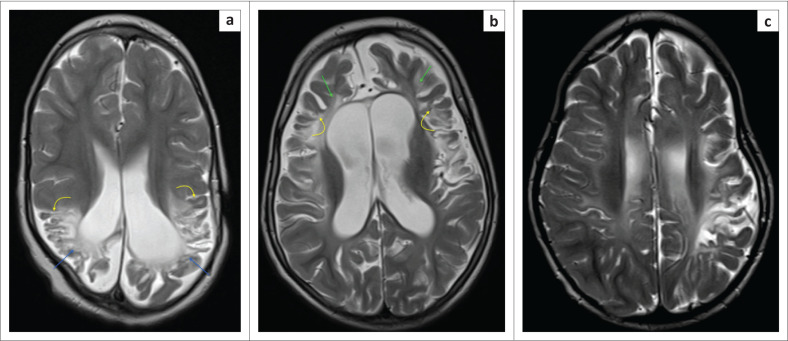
Axial T2-weighted magnetic resonance images demonstrating severe post-hypoxic injury because of partial prolonged ischemia in three different patients. Note the spongiosis of the parietal lobes (blue arrows) (a) and frontal lobes (green arrows) (b) associated with ulegyria (yellow arrows) and secondary ventriculomegaly. (c) The level of centrum ovale demonstrating asymmetric hypoxic ischemic brain injury changes, greater in the left frontal and parietal lobes. This latter pattern of asymmetric involvement is not an uncommon finding.
